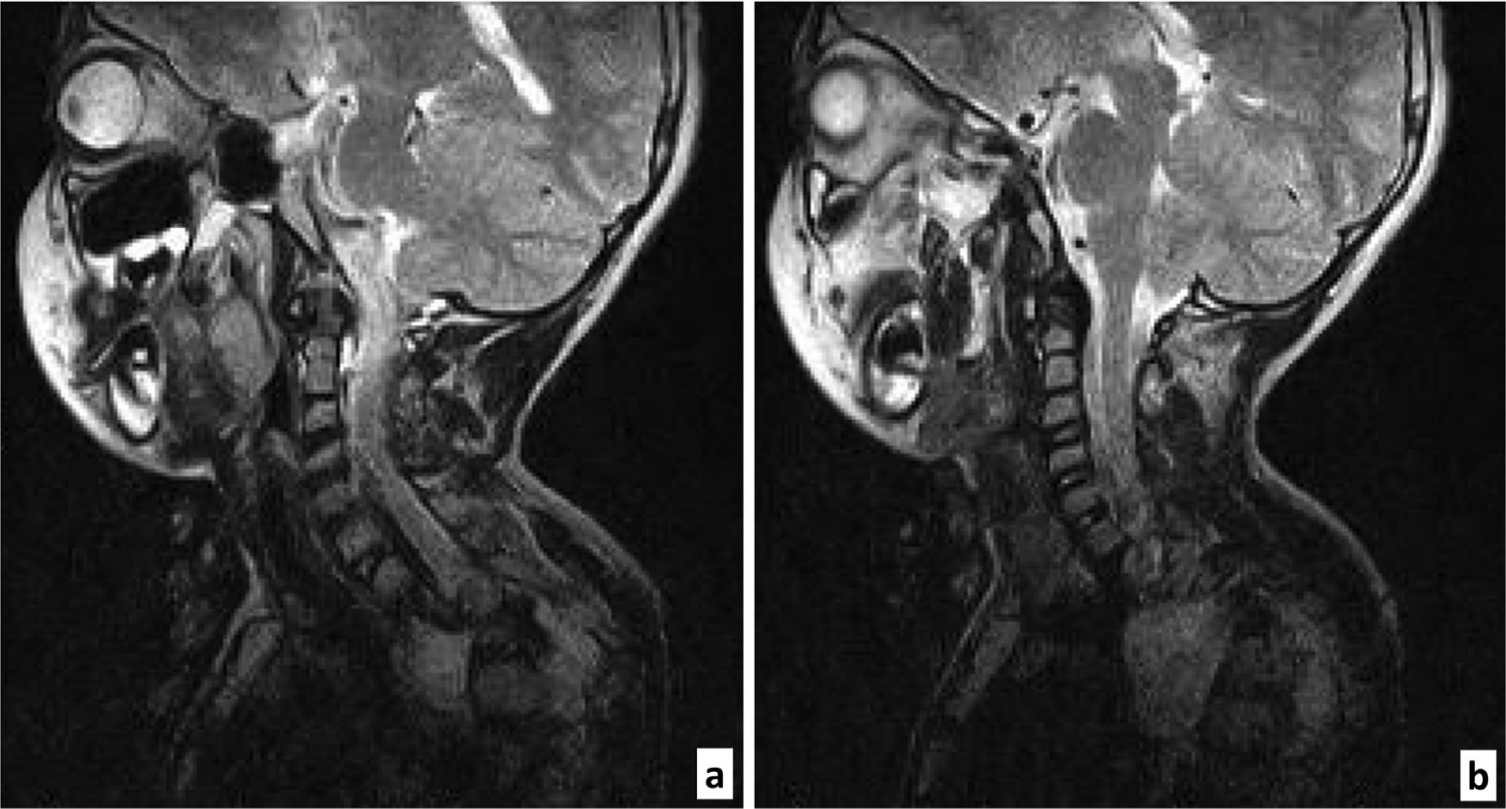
MRI craniocervical junction. Midsagittal (A) and right paramedian (B) slices in T2WI FSE. No engagement seen nor any abnormality of the posterior fossa.
